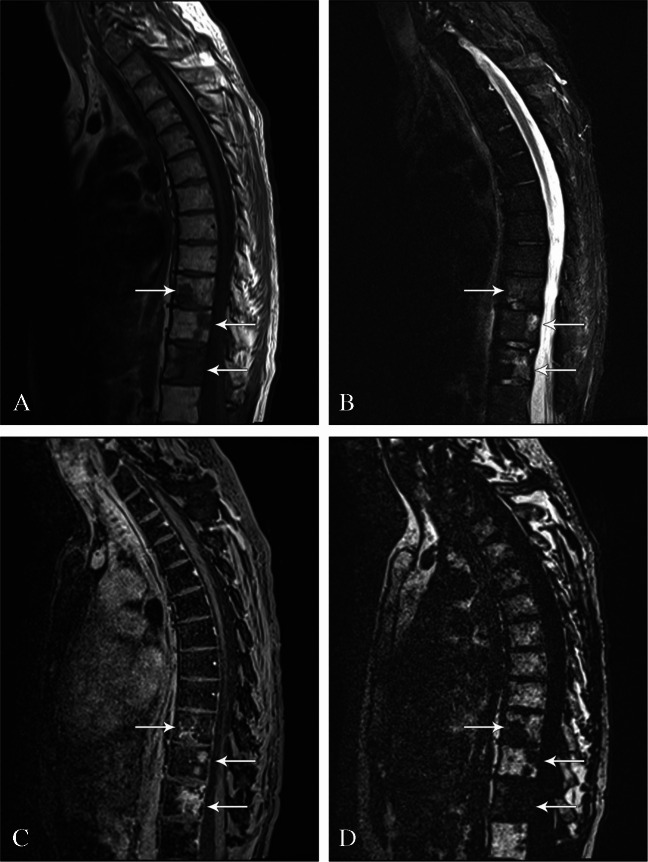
38-year-old male with metastatic Ewing sarcoma. Sagittal T1 (A) and STIR (B) and T1 Dixon volumetric interpolated breath-hold examination (VIBE) post-contrast Water (C) and Fat (D) MRI images demonstrate metastases of the T10-12 vertebra (arrows) that enhance and replace the normal fatty bone marrow
